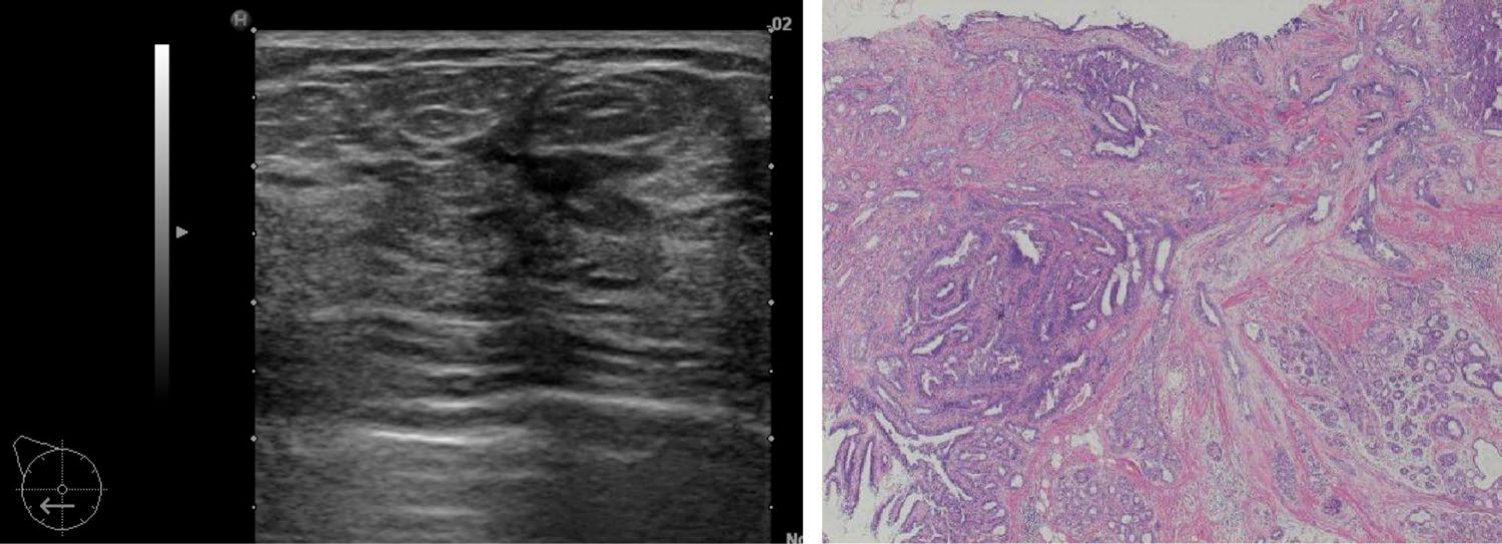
Case of radial sclerosing lesion. Ultrasound shows the architectural distortion
